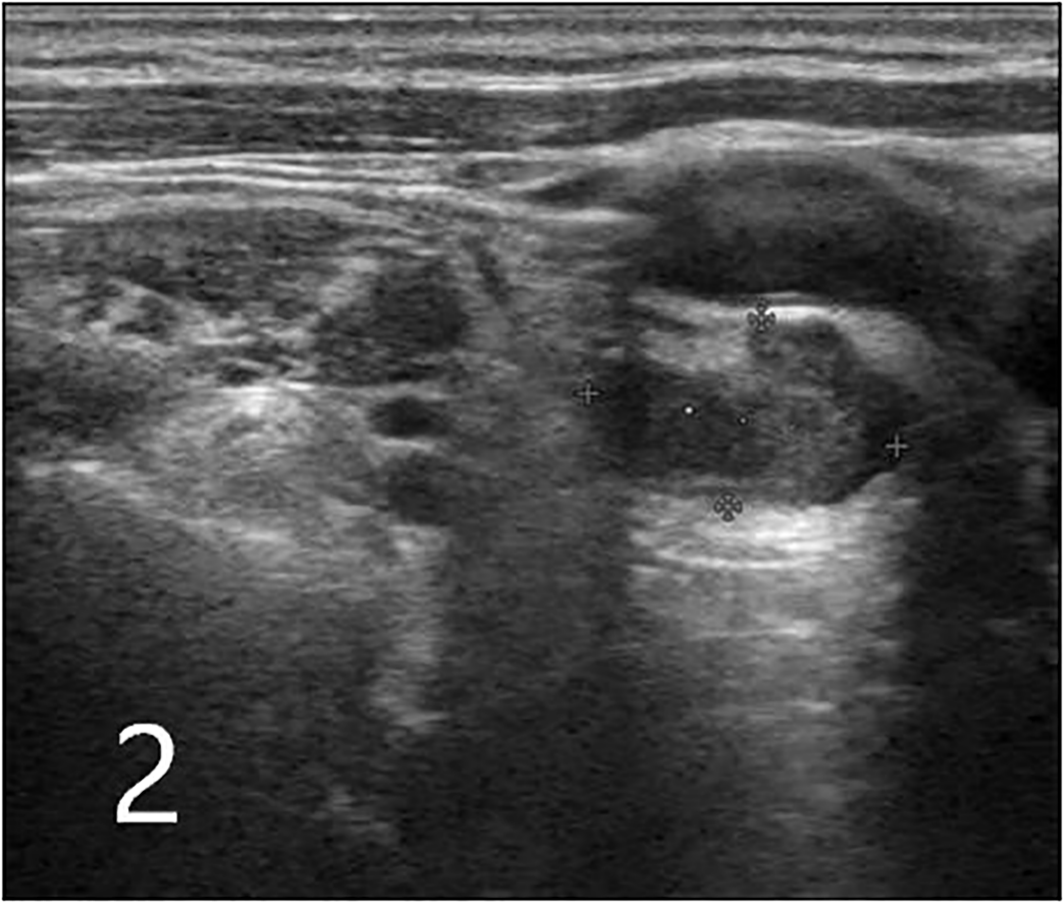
Ultrasound image of an enlarged inhomogeneous hypoechoic cervical lymph node with irregular morphology in level IV on the right side (indicated by arrows; plus sign indicates the measurement of lymph node size, which was 1.5 cm × 0.9 cm).
